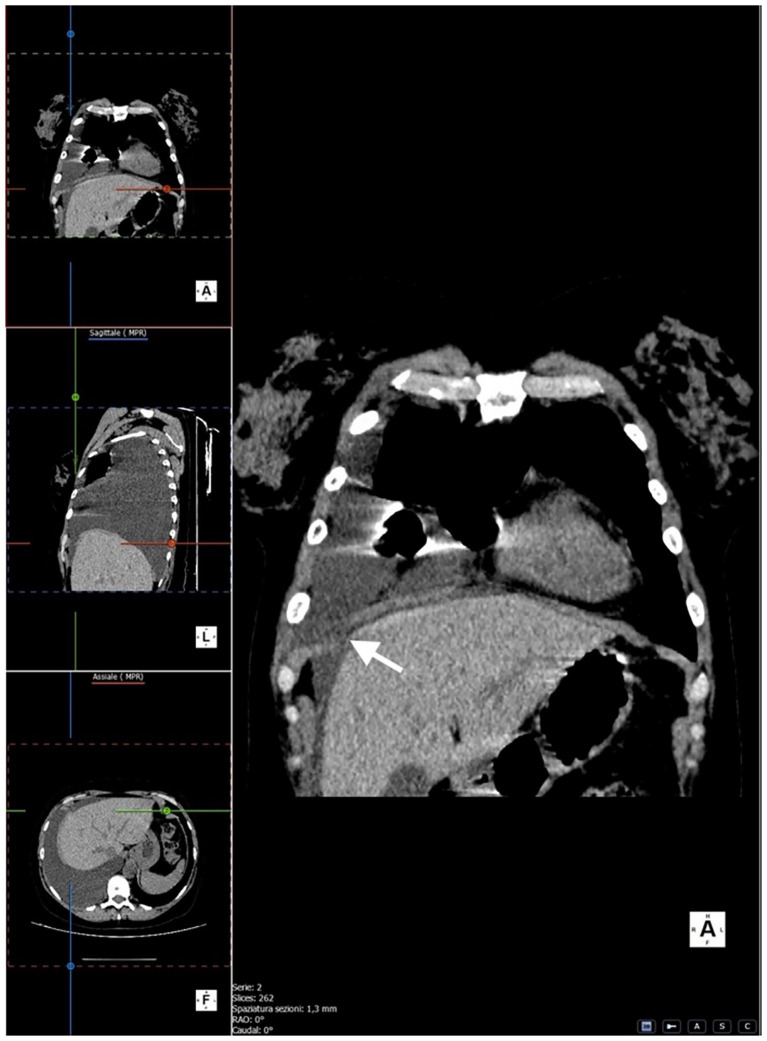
Coronal chest CT showing a suspected diaphragmatic discontinuity (white arrow).
